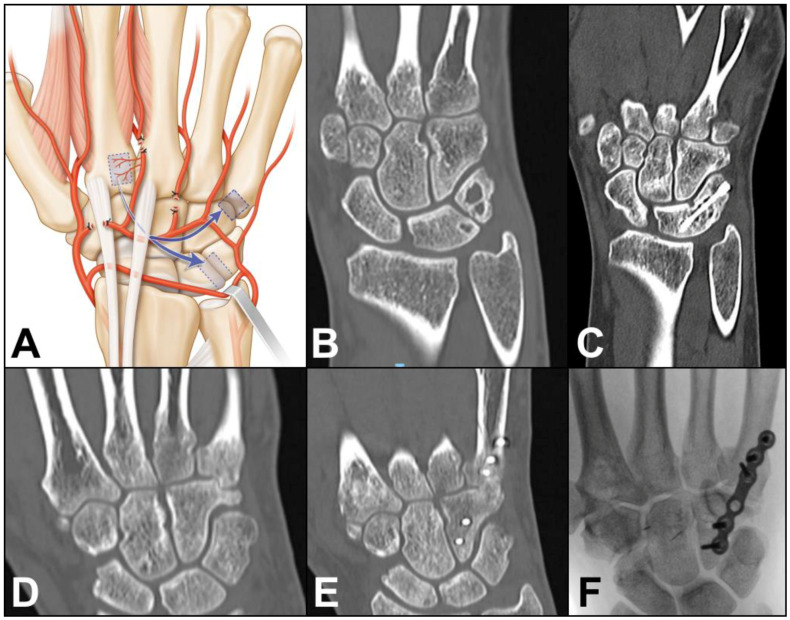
(A) Arterial anatomy, ligation of several arterial branches is necessary to allow the graft to reach the ulnar side of the carpus; Case 2. (B) preoperative CT-scan showing lunotriquetral arthritis; (C) postoperative CT-scan confirming fusion of the joint. Case 3. (D) preoperative CT-scan showing fifth carpometacarpal arthritis; (E,F) postoperative CT-scan and X-ray confirming fusion of the joint.
